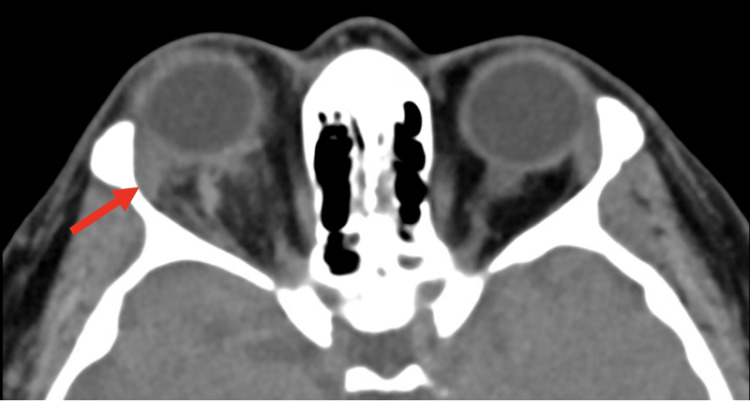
Axial non-contrast CT image showing a hyperdense retrobulbar infiltration and proptosis of the right eye (red arrow)
